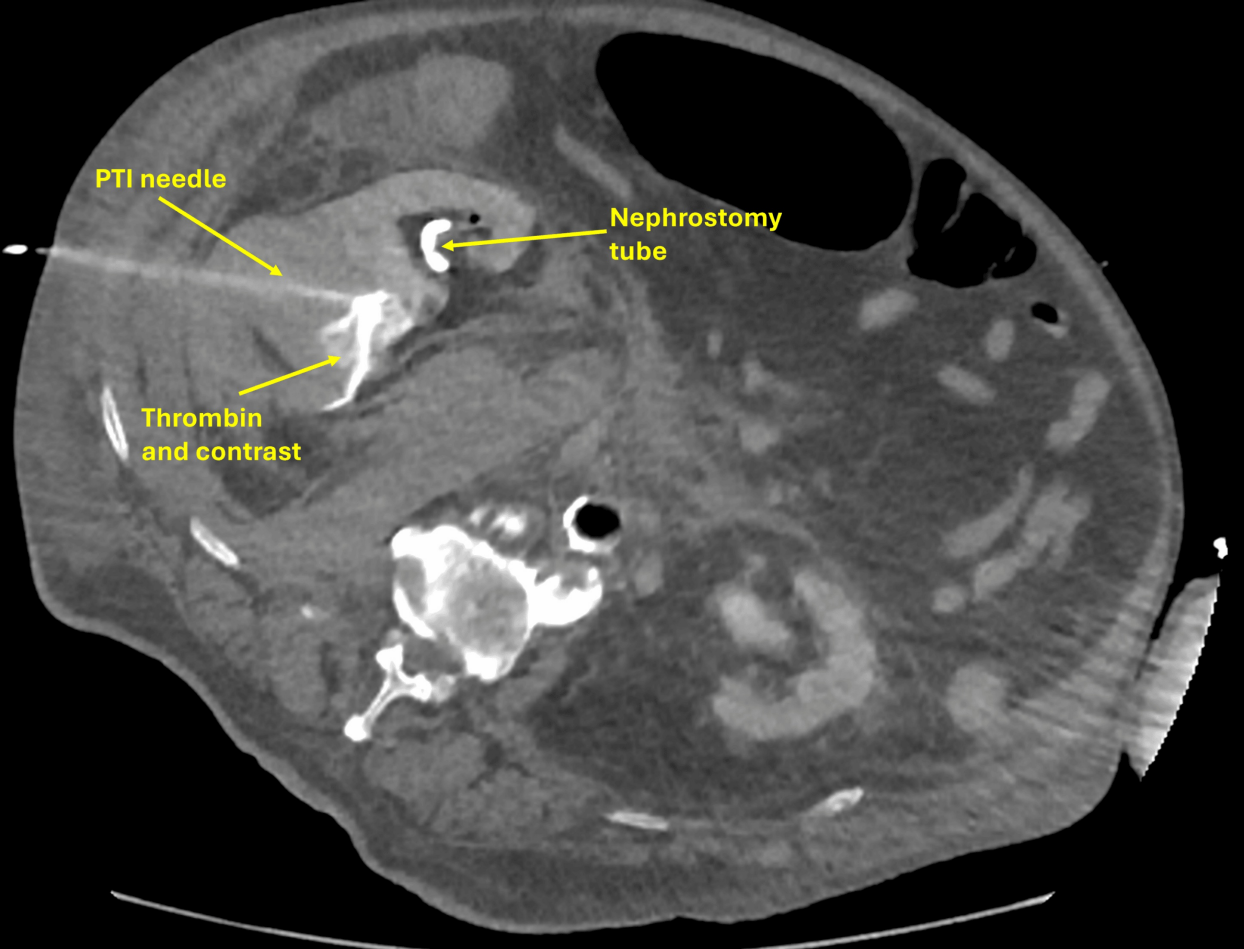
CT-guided thrombin injection into the right perinephric hematoma at the nephrostomy site. PTI: percutaneous thrombin injection.
